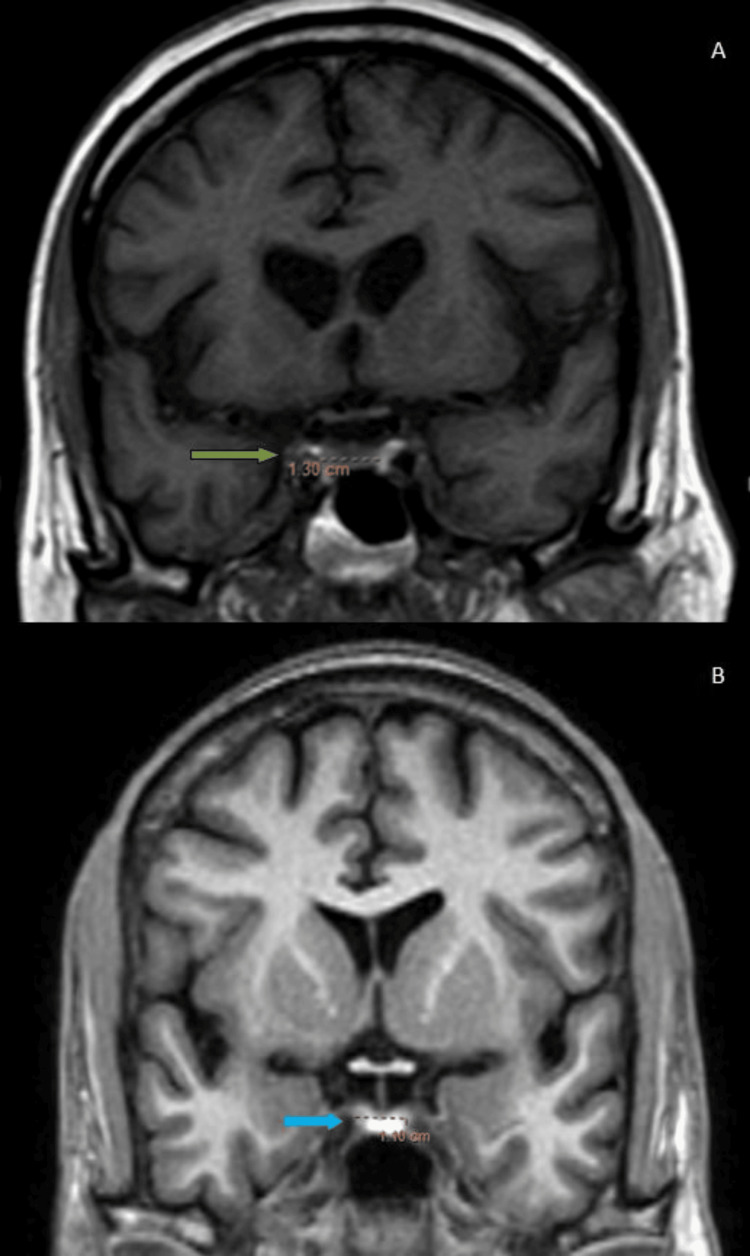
(A, B): MRI of the sella turcica. (A) T1 mid-coronal: the pituitary gland in a 35-year-old male shows a transverse dimension of 13 mm (green arrow). (B) T1 mid-coronal: the pituitary gland in a 45-year-old female shows a transverse dimension of 11 mm (blue arrow)
